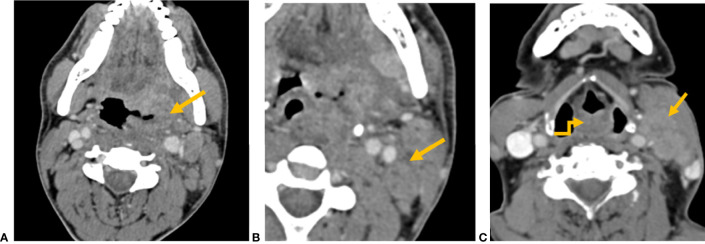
CT axial image shows (A) left tonsillar fossa SCC (yellow arrow) and (B) metastatic left level II node showing capsular irregularity and surrounding fat stranding (yellow arrow), representing extranodal extension. (C) CT axial image shows an ill-defined mass involving the aryepiglottic fold (yellow elbow arrow). Metastatic left level II node with gross muscle invasion represents extranodal extension (yellow arrow).
